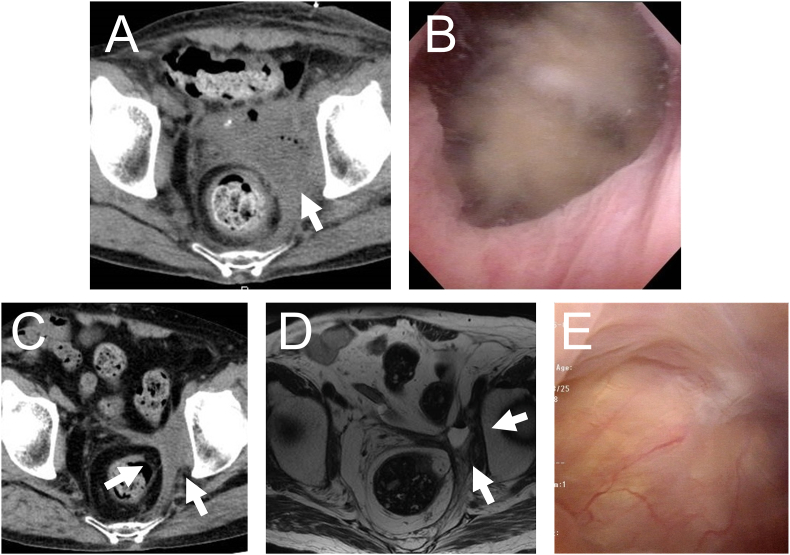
Imaging and cystoscopic findings during treatment with pembrolizumab. (A) CT scan during pembrolizumab treatment. The arrow indicates partial response. (B) Cystoscopy. Necrotic tissue filling the bladder is observed with the cystoscope. (C) CT scan 2 years after discontinuation of treatment. The arrow indicates atrophic bladder and pooling of fluid in the diverticulum. (D) MRI image 2 years after discontinuation of treatment. The arrow indicates atrophic bladder and pooling of fluid in the diverticulum. (E) Cystoscopy 2 years after discontinuation of treatment.
